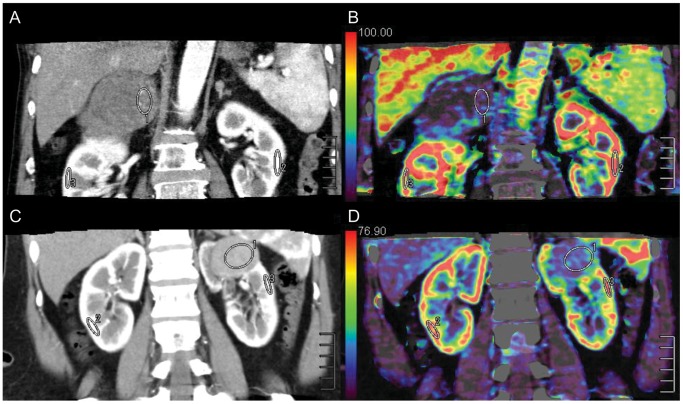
ROIs (ROI 1, ROI 2, and ROI 3) were drawn in the tumor and in healthy ipsi- and contralateral renal cortex. ROI 1 was the ROI of tumor tissue. Papillary RCC (a) with the characteristics of hypo-vascularity (mean CT value = 67.4 HU) showed low perfusion values (mean Equiv BV = 14.1) (B), while chromophobe RCC (C) with a moderate blood supply (mean CT value = 85.0 HU) appeared low perfusion values (mean Equiv BV = 20.8) (D) too.
